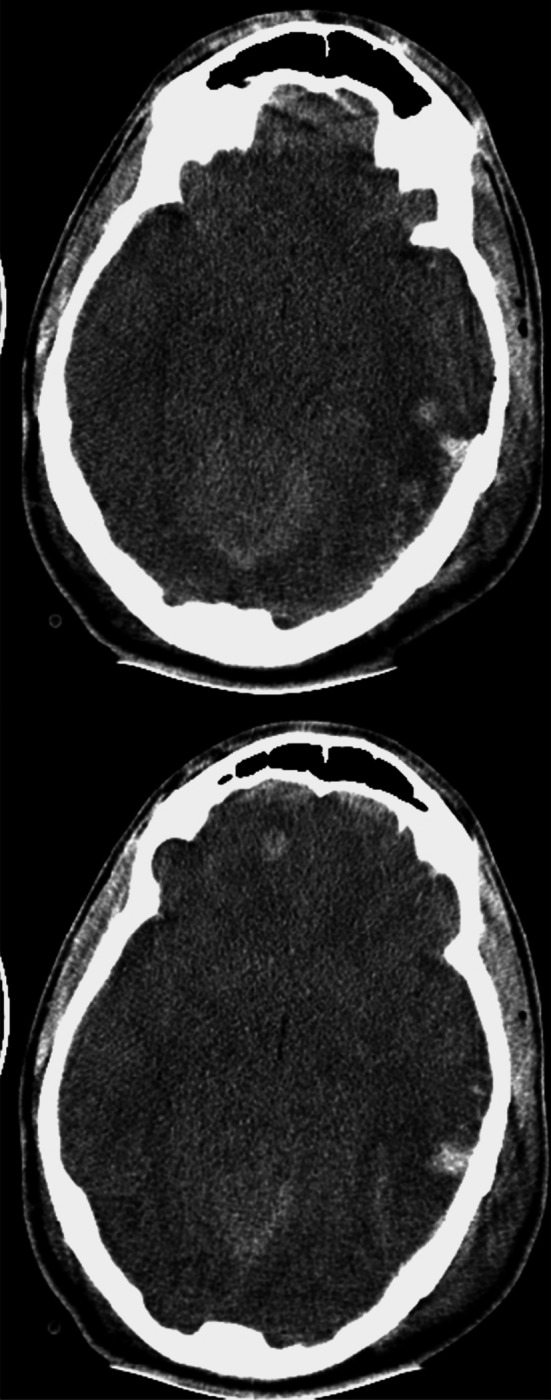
Axial CT scans showing non-penetrating blast overpressure effect on the brain. *Note* the generally swollen brain with loss of basal cisterns, slit-lke third ventricle, loss of *gray-white* differentiation and multiple hemorrhagic contusions
